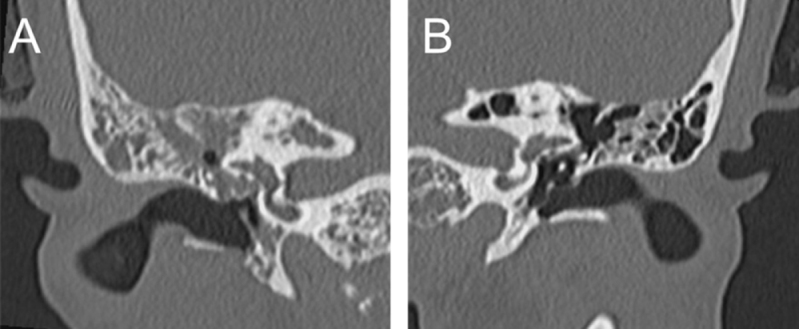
Axial temporal bone computed tomography. (A) Right side: Soft tissue opacity was observed in the tympanic and mastoid cavities. (B) Left side: Adequate aeration was preserved in the tympanic and mastoid cavities.
